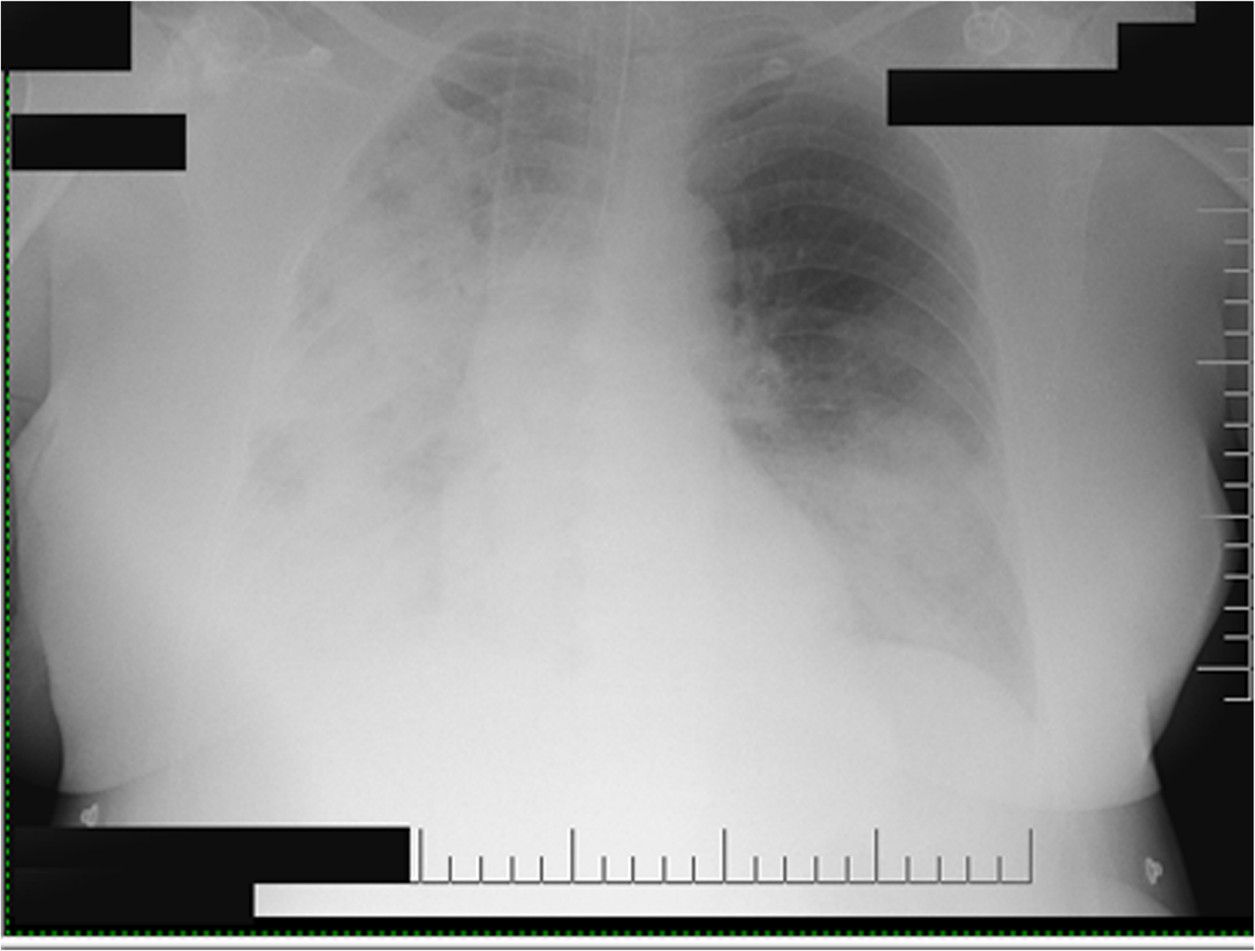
Chest X-rays at the moment of ICU admission, after tracheal intubation and mechanical ventilation initiation. Alveolar consolidation of the entire right lung and the lower lobe of the left lung are seen
